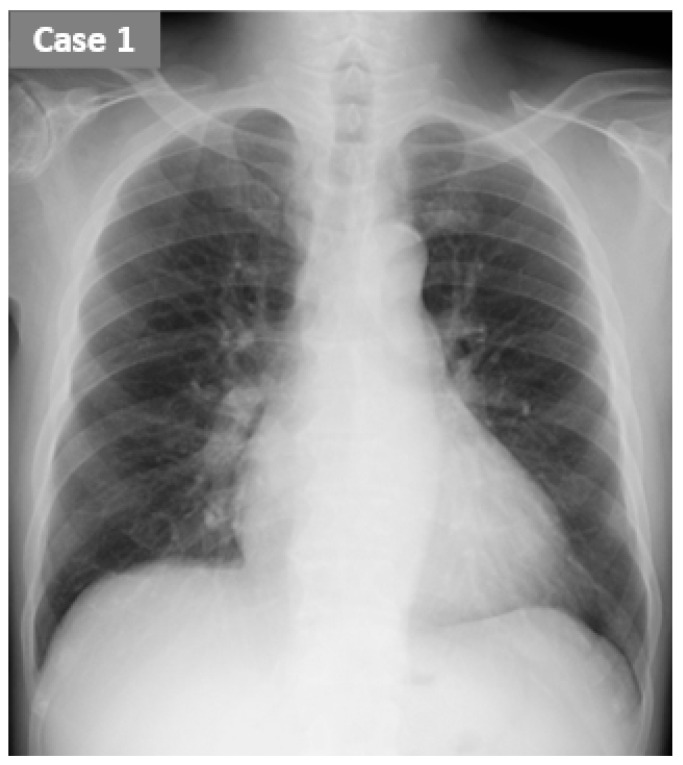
The chest X-ray just before hemodialysis in case 1.
